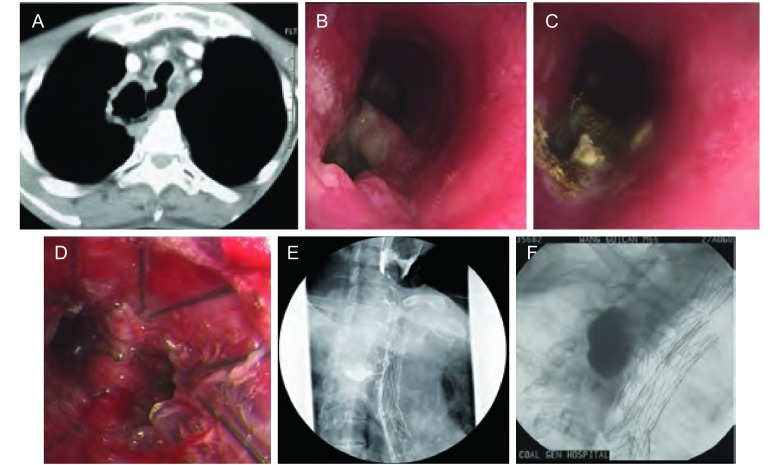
食管癌术后合并食管气管瘘和食管纵隔瘘的综合治疗。A：CT可见气管与食管相通；B：气管镜可见气管下端膜部2 cm×1.5 cm瘘口；C：用APC将瘘口周围的肿瘤烧灼；D：置入Y型气管支架将瘘口封闭；E：食管碘油造影显示气管食管瘘口封闭，但造影剂流到纵隔内；F：放置食管支架，将食管纵隔瘘口封闭。 Multiple modalities for the treatment of ERF and esophagomediastinal fistula in a patient with esophagus carcinoma postesophagostomy. A: CT showed that there is a channel between esophagus and trachea; B: Bronchoscopy showed that a fistula (2 cm×1.5 cm) is located in the back of trachea; C: Tumor around trachea fistula was ablated by APC; D: Y-shaped covered stent was inserted to seal the fistula; E: Esophageal hysterosalpingography showed that esophageal-trachea fistula was completely sealed, but contrast agent was drainage to mediastinal which suggested that esophageal-mediastinal fistula existed; F: Esophagus stent was inserted to seal the esophageal-mediastinal fistula.
